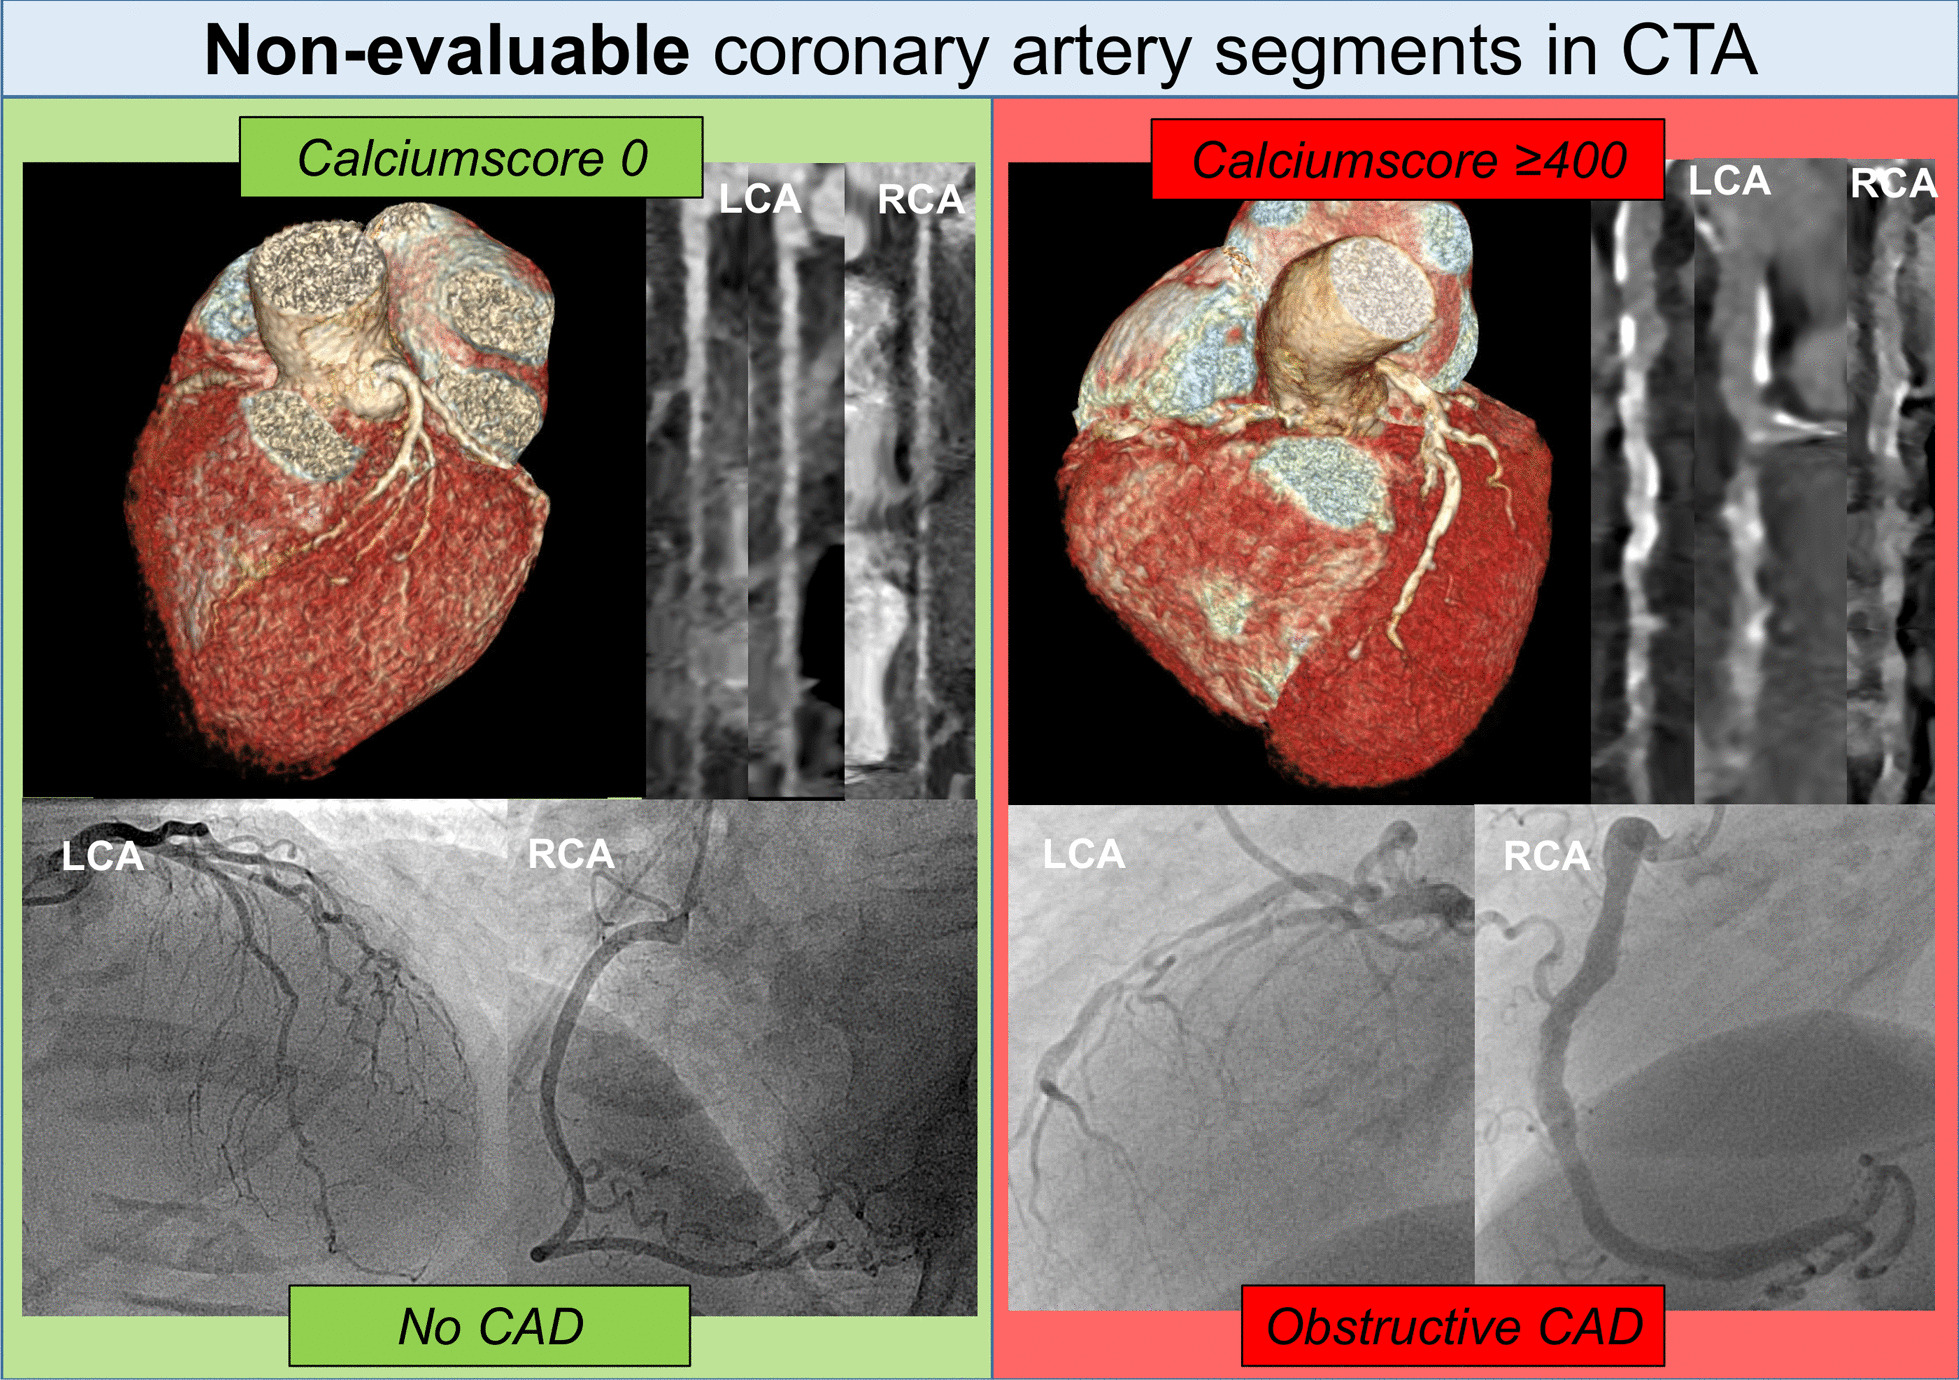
Examples of patients undergoing pre-TAVI CT with “non-evaluable” coronary artery segments and consecutive application of CACS. On the left side a patient is presented where pre-TAVI CTA coronary segments were non- evaluable, however CACS was 0 and no obstructive CAD was confirmed by invasive QCA. On the right hand side, a patient is presented, where CTA was non-evaluable, but CACS was above 400 and invasive QCA confirmed obstructive CAD (i.e. high grade stenosis in the LAD/1.diagnoal branch). *CACS* coronary artery calcium score, *CAD* coronary artery disease, *CTA* computed tomography angiography, *RCA* right coronary artery, *LCA* left coronary artery
